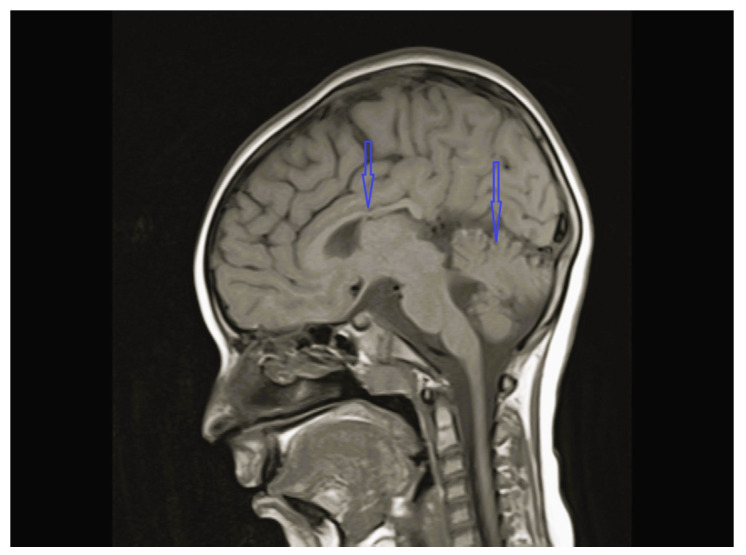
Brain MRI of case 3 revealing cerebellar and corpus callosum hypoplasia.
